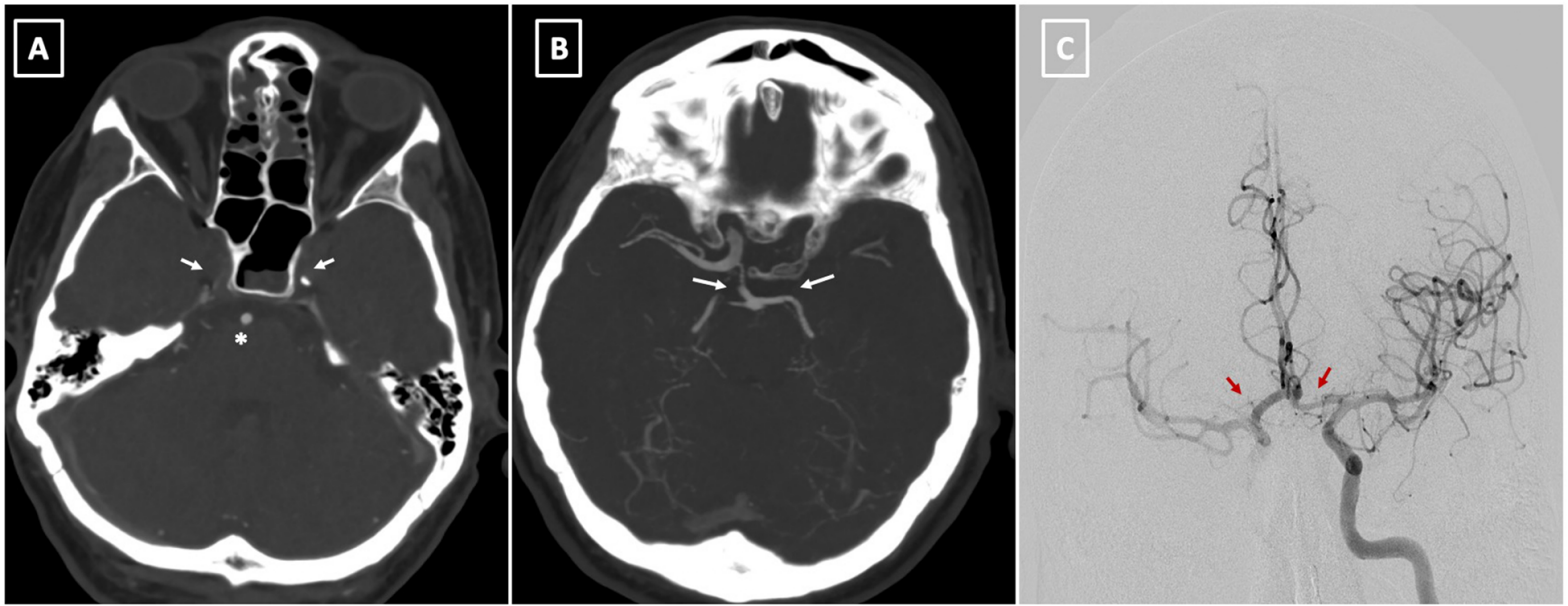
(A) preoperative CT angiography at level of skull base demonstrates bilateral occlusion of internal carotid arteries (arrow) with a patent basilar artery (asterisk). (B) The Maximal Intensity Projection (MIP) reconstruction highlights the distal filling of the right internal carotid artery through the patent right posterior communicating artery (Pcom). Notably, the left Pcom is absent, resulting in insufficient collateral flow to the left cerebral hemisphere. (C) This image shows successful reperfusion of both cerebral hemispheres following the stent placement in the left common carotid artery (LCCA) (arrows).
